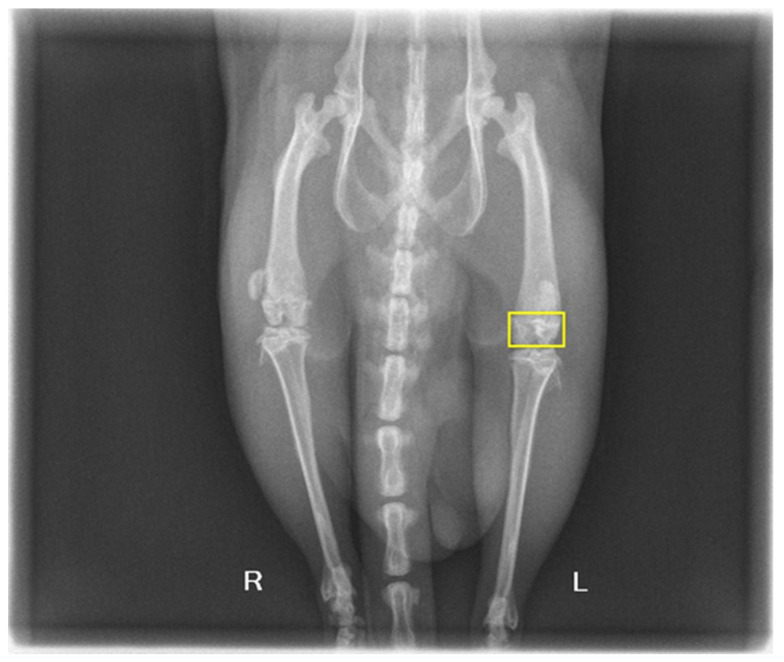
Postoperative X-ray of test subject with area of analysis highlighted.
